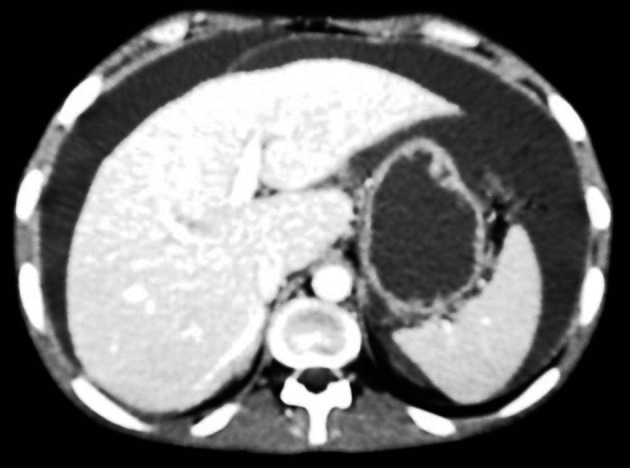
Ascites apperance on CT of the abdomen.
